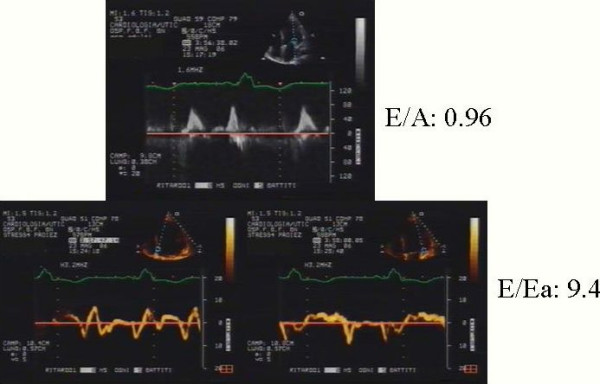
Transmitral LV filling pattern (up); mitral annulus pulsed Tissue Doppler velocity at septum (left panel) septal (down left) and lateral wall (down right) corner.
